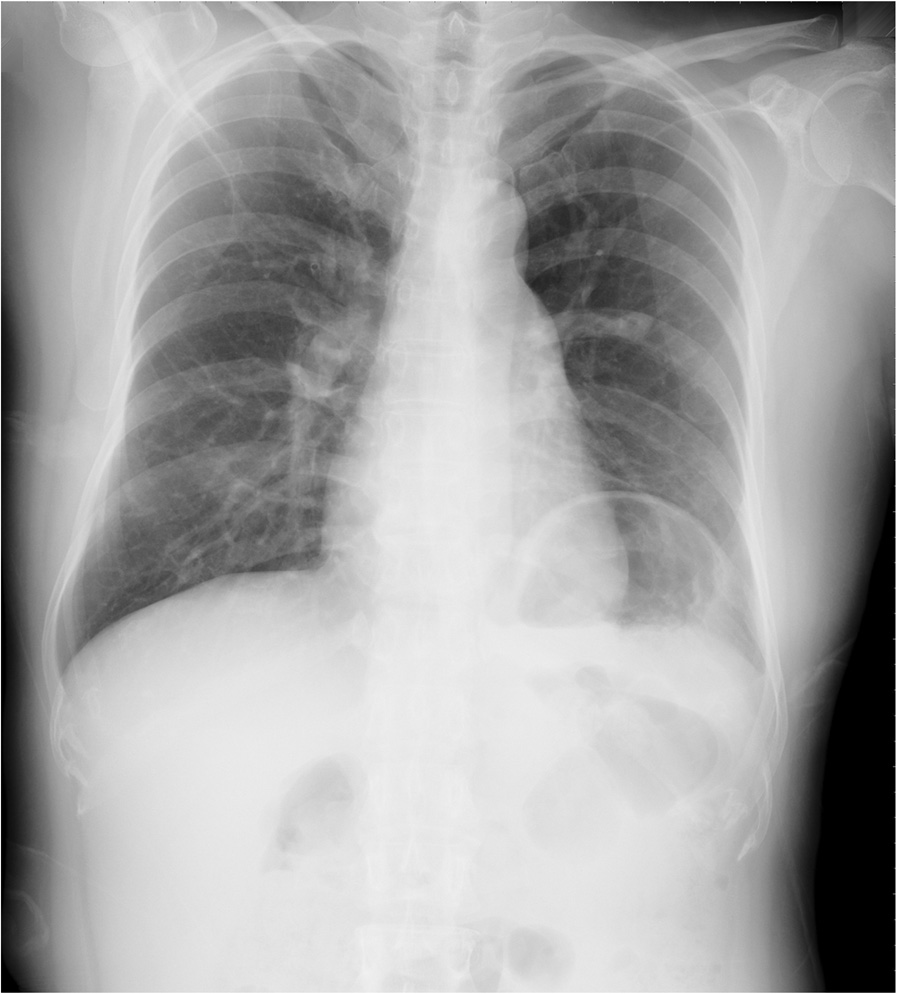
Chest radiograph of a 44-year-old woman showed an abnormally elevated diaphragm on the left side. Subsequent CT scan confirmed the diagnosis of eventration.
